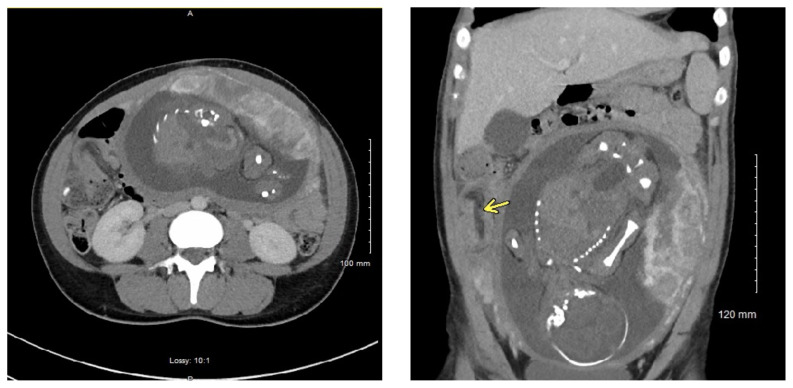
Axial CT Video Link: https://youtu.be/iPkbvEJ1Vms Coronal CT Video Link: https://youtu.be/29GMR59ohnw
